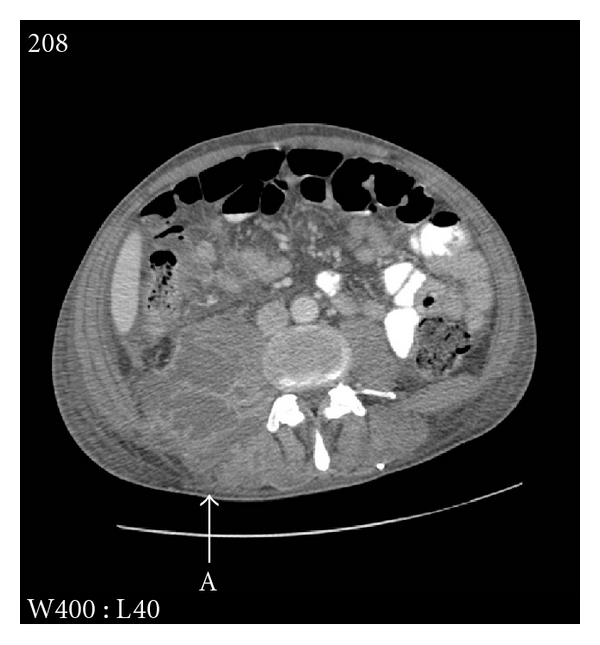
CT, axial view. The loculated right-sided psoas abscess extends to the skin surface (A).
